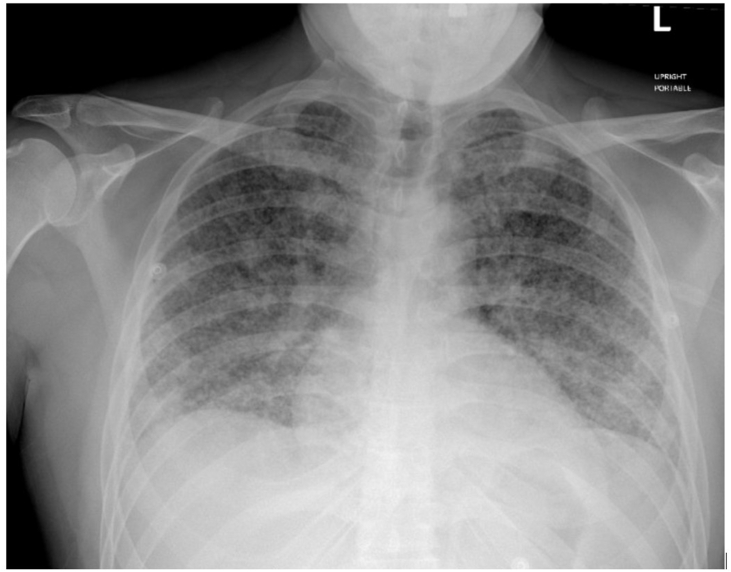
Chest radiograph showing diffuse miliary pattern of micronodularity in both lungs.
